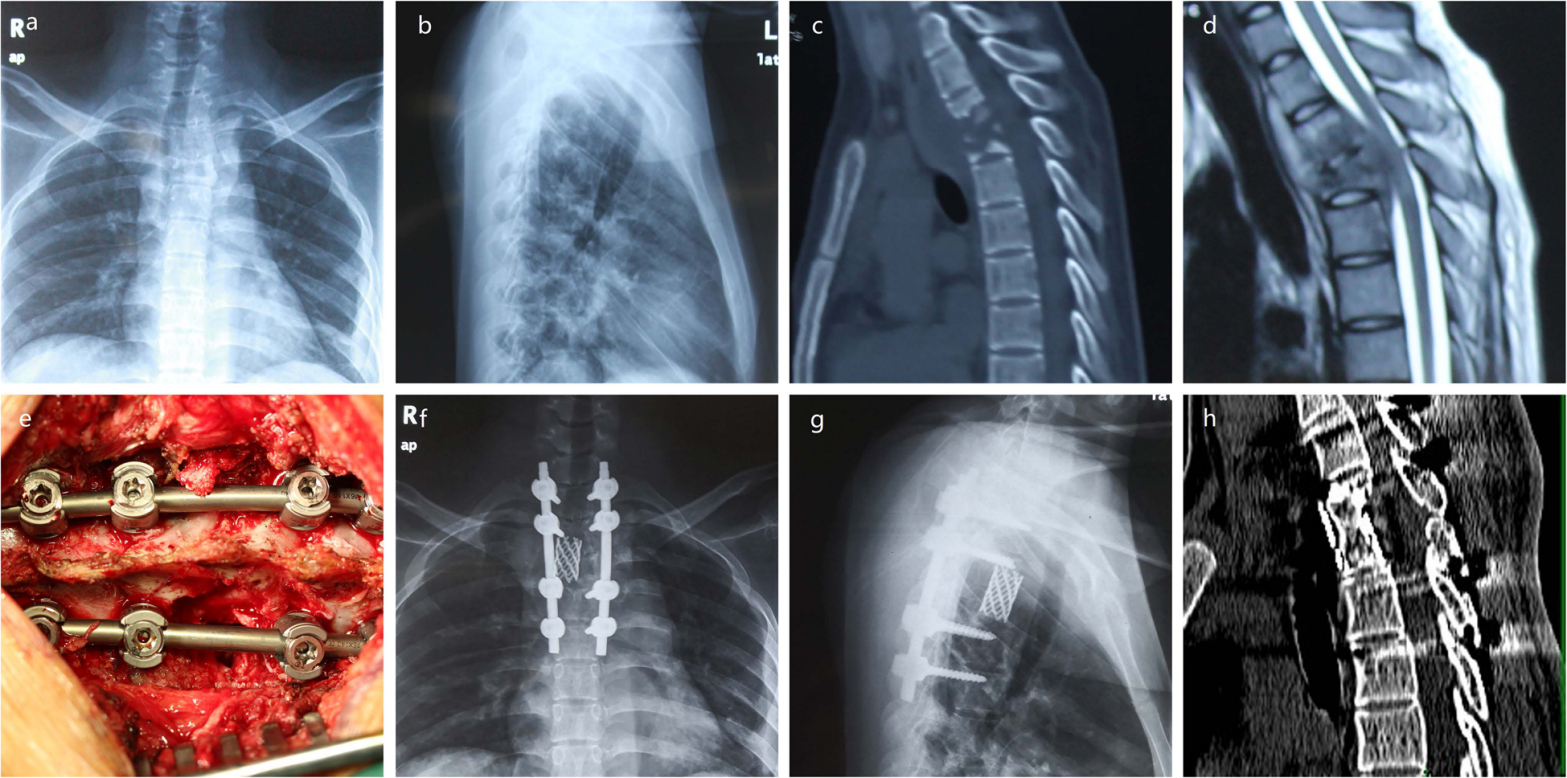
A 26-year-old woman was diagnosed with thoracic tuberculosis after a 6 months history of intermittent back pain and numbness in both lower limbs. a-b preoperative X-ray showed that T2–3 tuberculosis with the vertebral body destroyed. c-d preoperative CT and MRI showed T2–3 vertebral body destruction with paravertebral abscess and spinal canal abscess with cord compression. e intraoperative image showed one-stage posterior debridement, allograft bone graft using titanium mesh, posterior instrumentation, and fusion. f-g postoperative X-ray before discharge showed well-positioned titanium mesh and posterior instrumentation. h CT scan at final follow-up (5 years) showed good bony fusion, correctly placed titanium mesh, and posterior instrumentation
